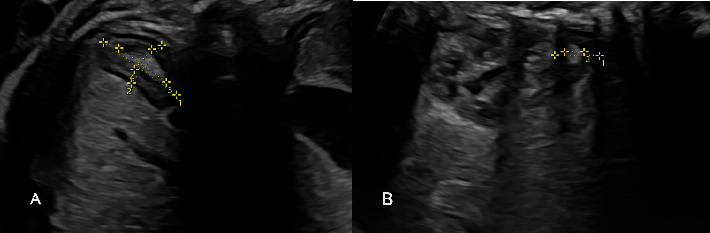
Two-dimensional fetal adrenal gland ultrasonography. (A) Axial plane: length (1) and width (2) of the WAG and length (3) and width (4) of the FZ. (B) Sagittal plane: depth of the WAG (1) and depth of the FZ (2). Abbreviations: FZ, fetal zone; WAG, whole adrenal gland.
